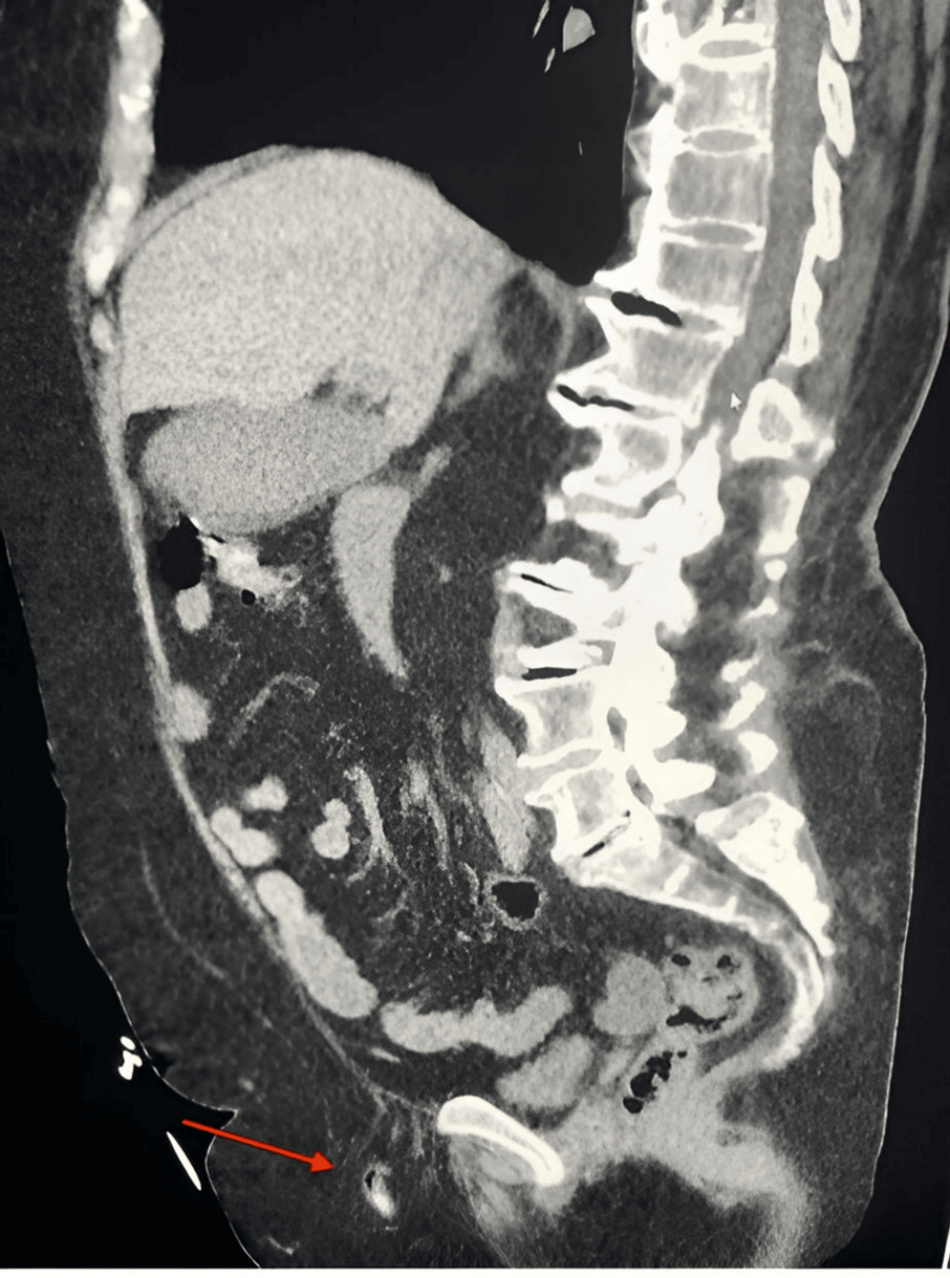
The sagittal CT of the abdomen shows a herniated appendix without any inflammation.
